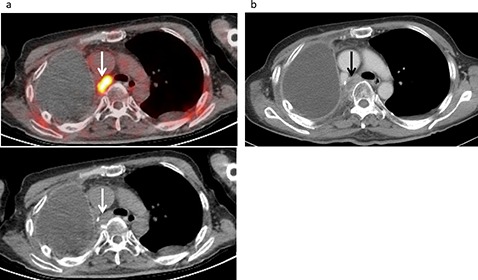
Lymph node recurrence developed in a 61-year-old male following treatment for a malignant pleural mesothelioma, including neoadjuvant chemotherapy, right extrapleural pneumonectomy, and radiotherapy. (a) FDG-PET/CT and CT findings showed intense FDG uptake (SUVmax 11.9) in a swollen mediastinal lymph node (arrow), strongly suggesting nodal metastasis with a score of 5. (b) Contrast-enhanced CT revealed a swollen mediastinal lymph node (12×18 mm) (arrow), suggesting nodal metastasis with a score of 4.
